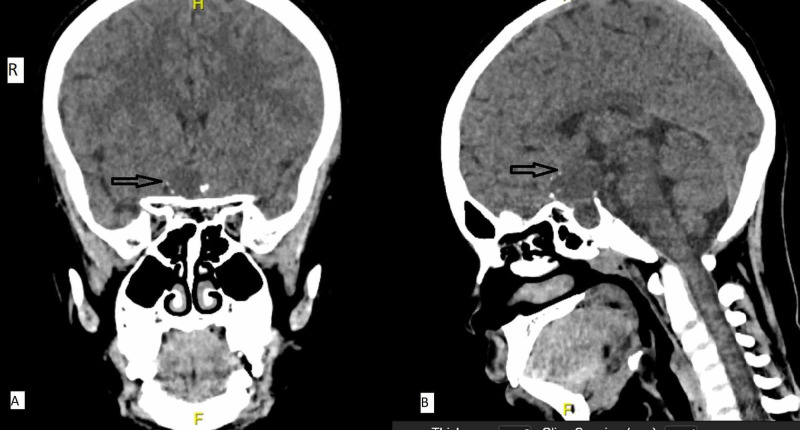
Computed tomography of the brain (A, coronal image and B, sagittal image) shows the tumour located superior to the pituitary gland (arrow).
